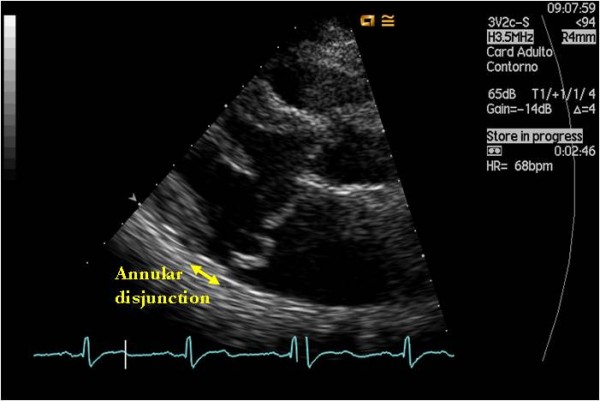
Parasternal long axis view of a myxomatous mitral valve showing significant disjunction of the posterior annulus.
